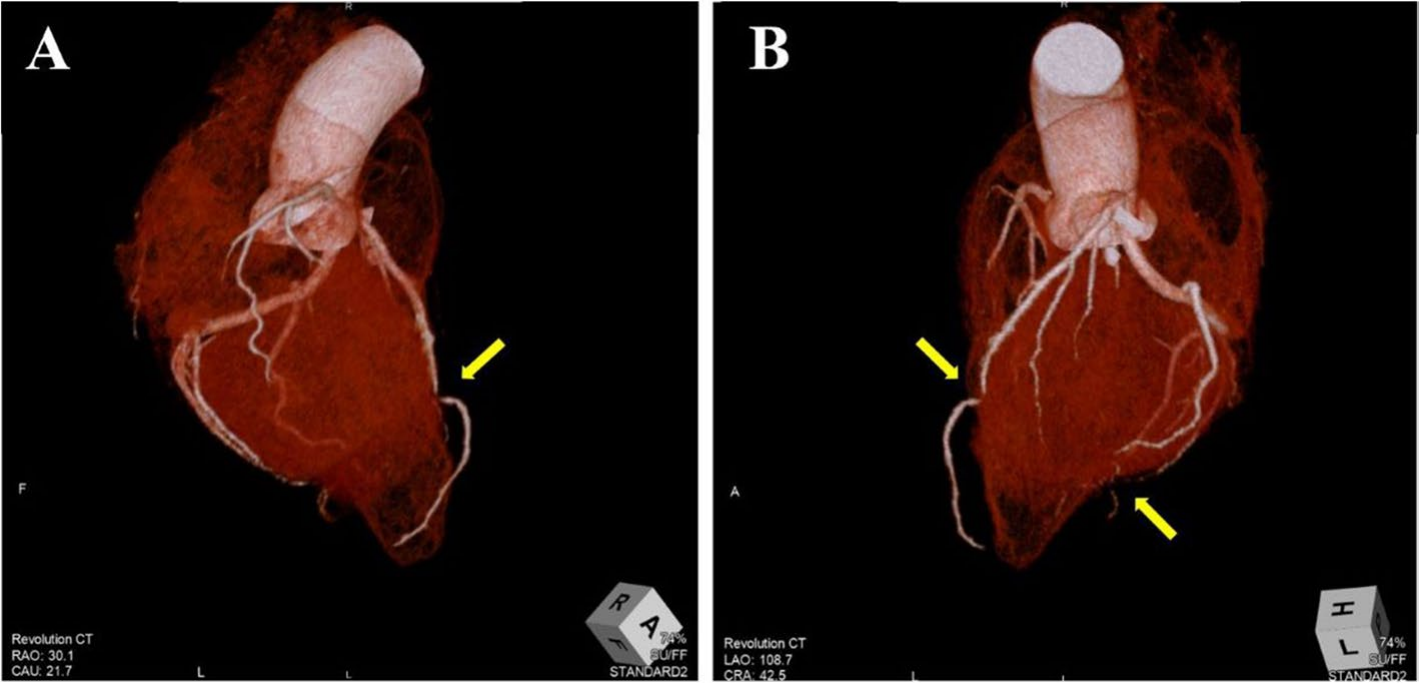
Computed tomography of the heart. Stenosis is observed at same sites identified on coronary angiography
